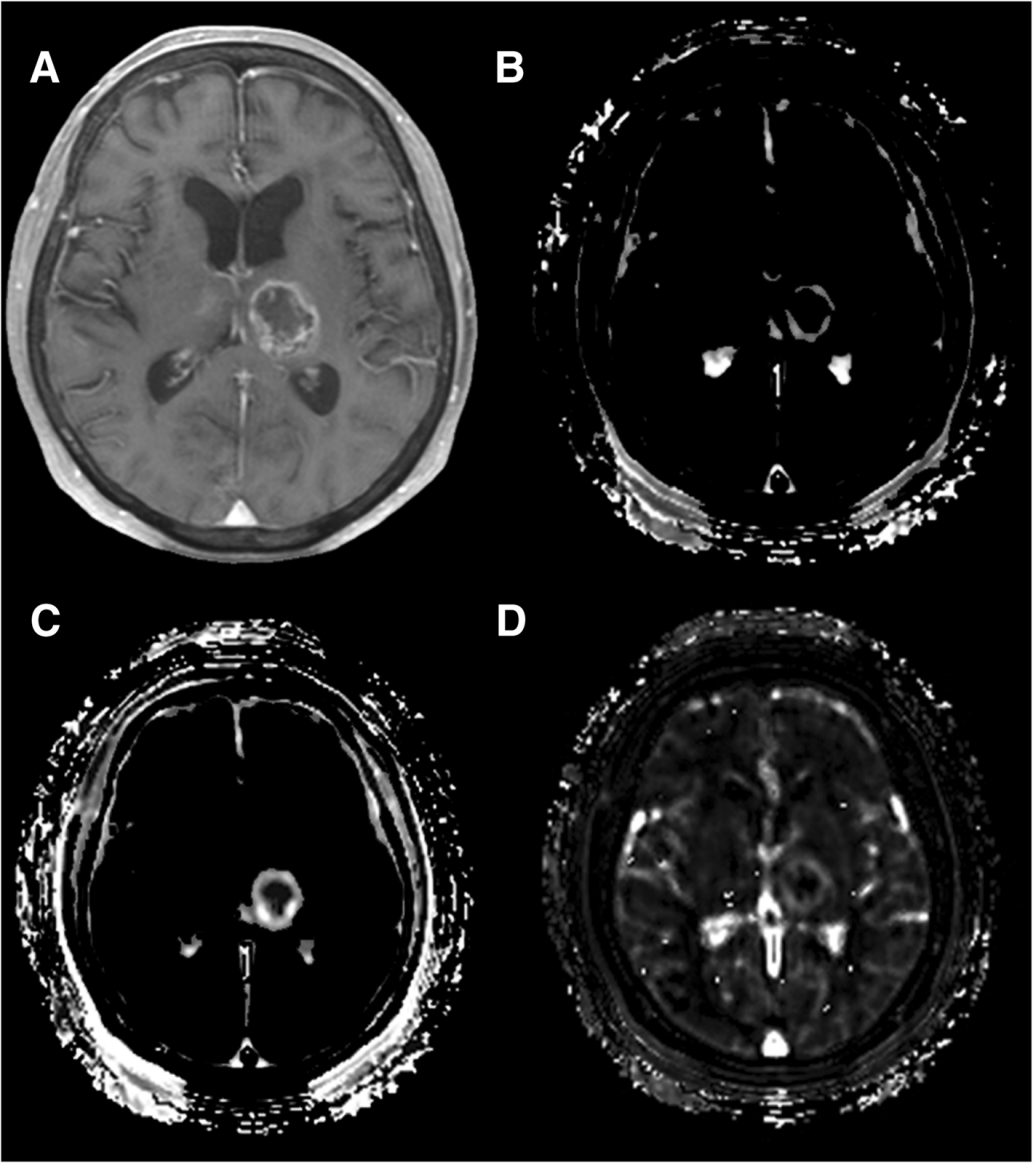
Magnetic resonance imaging of a glioblastoma. A, Axial post-contrast T1-weighted image shows a ringed enhanced lesion in the left thalamus and subtle enhancement in the right thalamus. B, C, and D, Three kinetic parametric maps show increased vascular permeability (B; *K*
